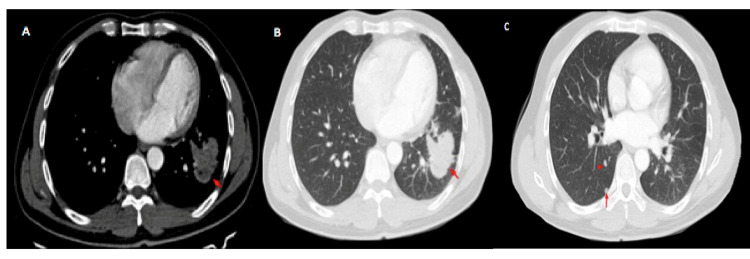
Axial CT images of left lower lobe lung tumoral process (A, B) and contralateral tumor nodules (C).
